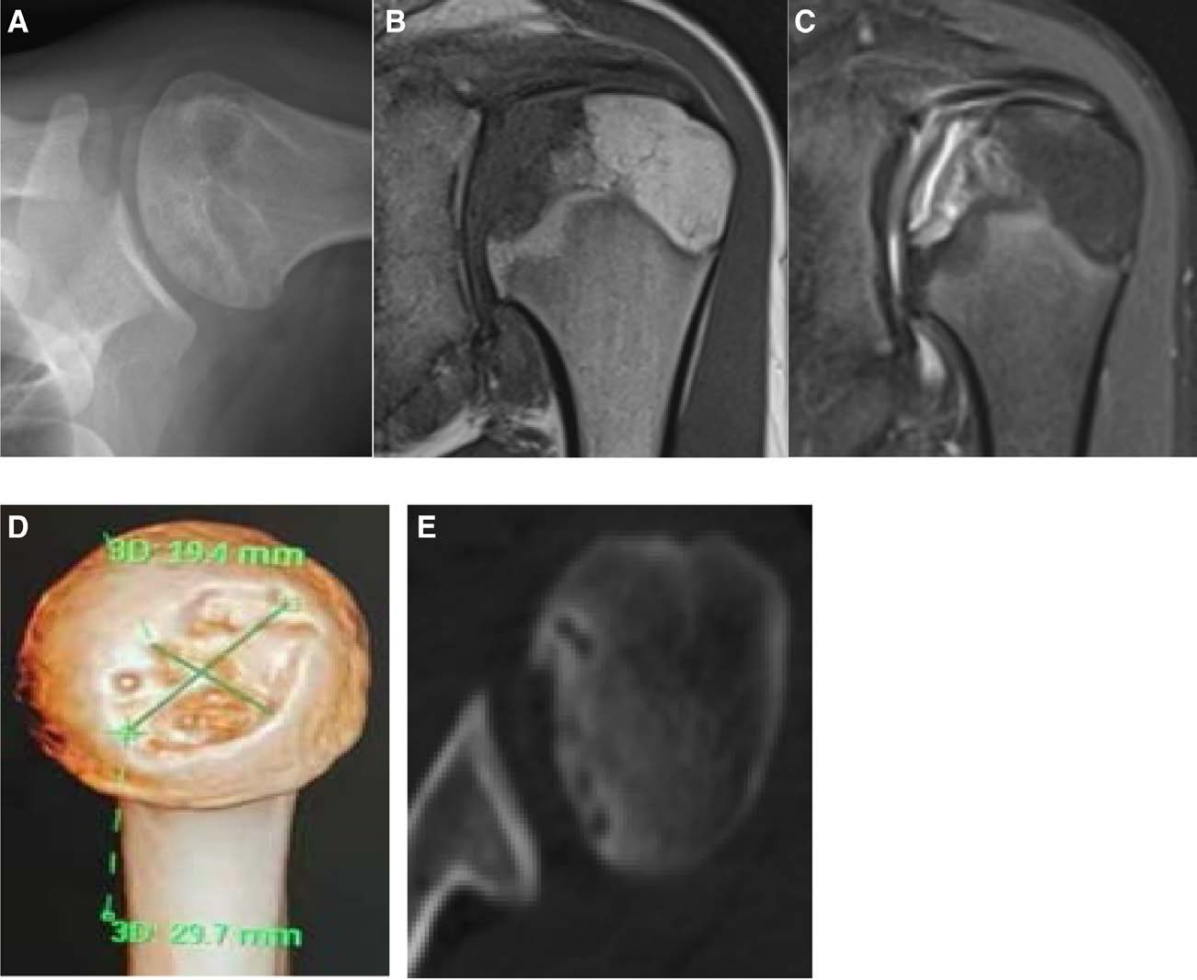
(A) X-ray AP of pre-operation. (B) MRI T1-weighted image. (C) MRI T2-weighted image. (D) 3-dCT scan. (E) CT scan cross section. CT = computed tomography, MRI = Magnetic resonance image.
